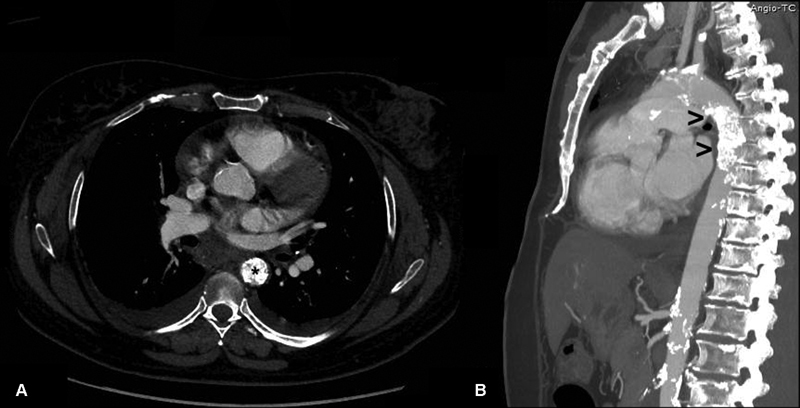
Computed tomography showing occlusion of the descending thoracic aorta (axial view in panel
